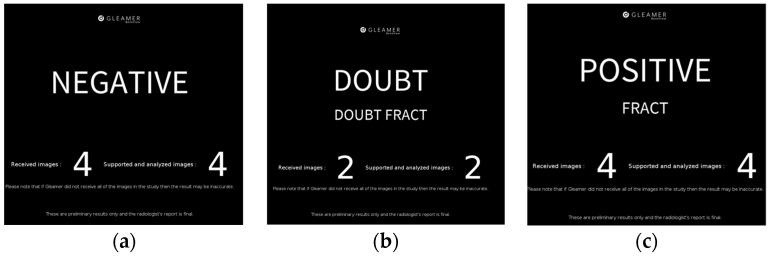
Result frames shown by the AI software delineating a case of exams without a fracture ((a), left), a possible fracture at 50–89% certainty threshold ((b), center) and a fracture at ≥90% certainty ((c), right). The number X-ray images analyzed in the exam is also shown.
